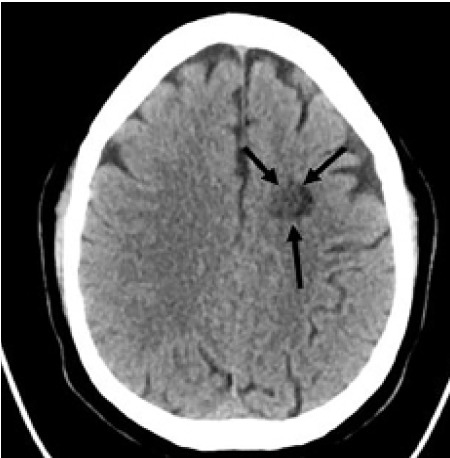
Computed tomography of the head without contrast in a 50-year-old male with dizziness. Black arrows identify an indeterminate 13-millimeter low density lesion in the left frontal lobe, further delineated by magnetic resonance imaging (Image 2).
